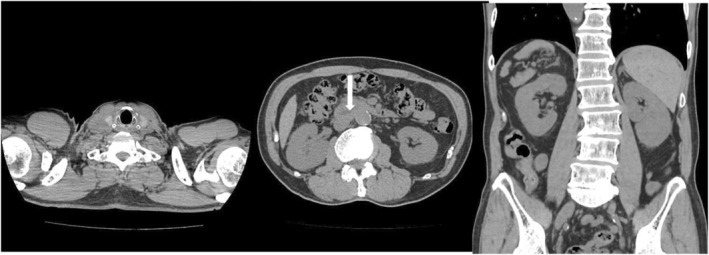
Twelve months after treatment, plain computed tomography shows that only slight enlargement of the abdominal para‐aortic lymph nodes is observed (⇩), and the right perinephric tumor and right supraclavicular lymph node continue to show clinical complete response.
